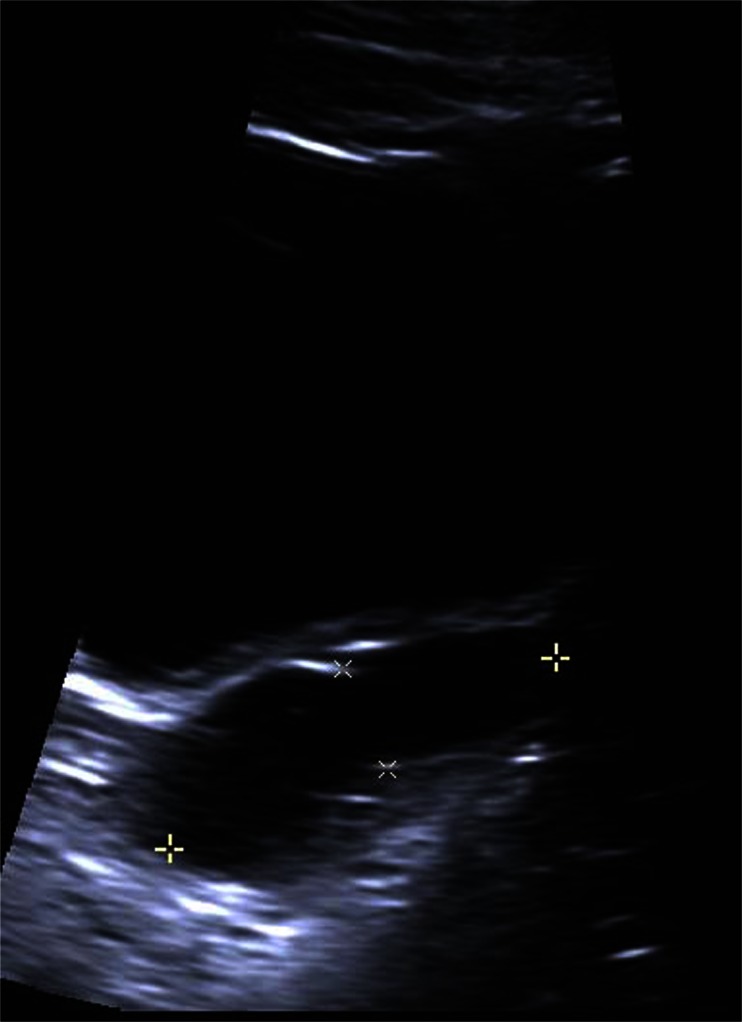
Initial ultrasound image (shows pathology)
